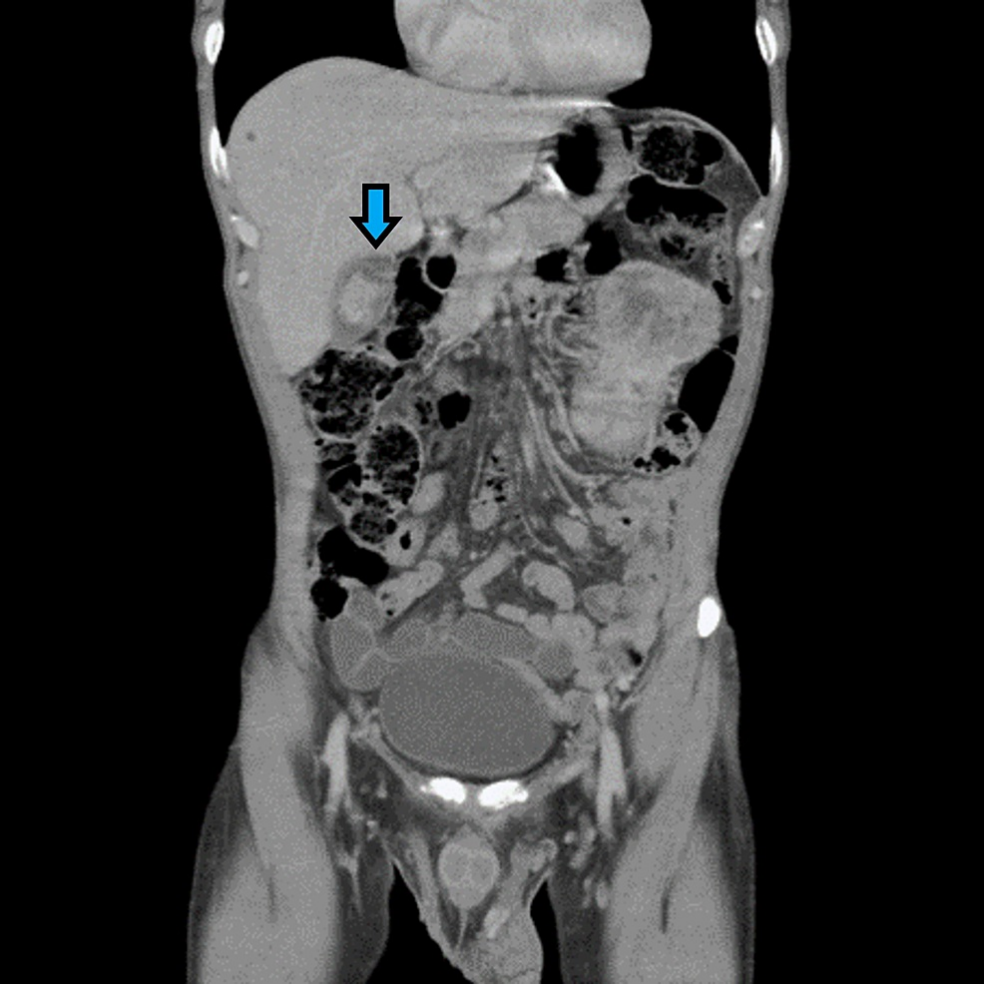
CT abdomen/pelvis with contrast demonstrating a soft tissue mass within the gallbladder in addition to a large left renal mass demonstrating both solid and complex cystic components.
